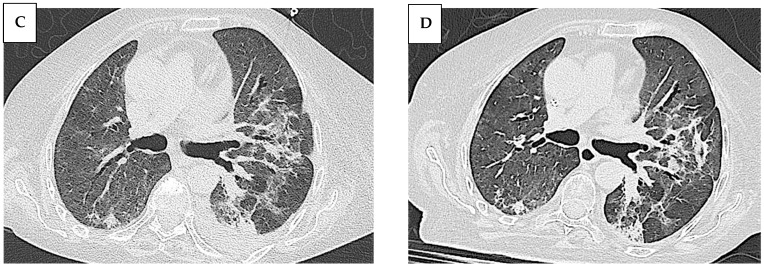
Alteration of radiologic ILD features due to pulmonary infections. Serial HRCT scan showing a reduction in areas of parenchymal consolidation before (A), during (B,C), and after (D) the immunosuppressive, antibacterial, antiviral, and antifungal therapies.
